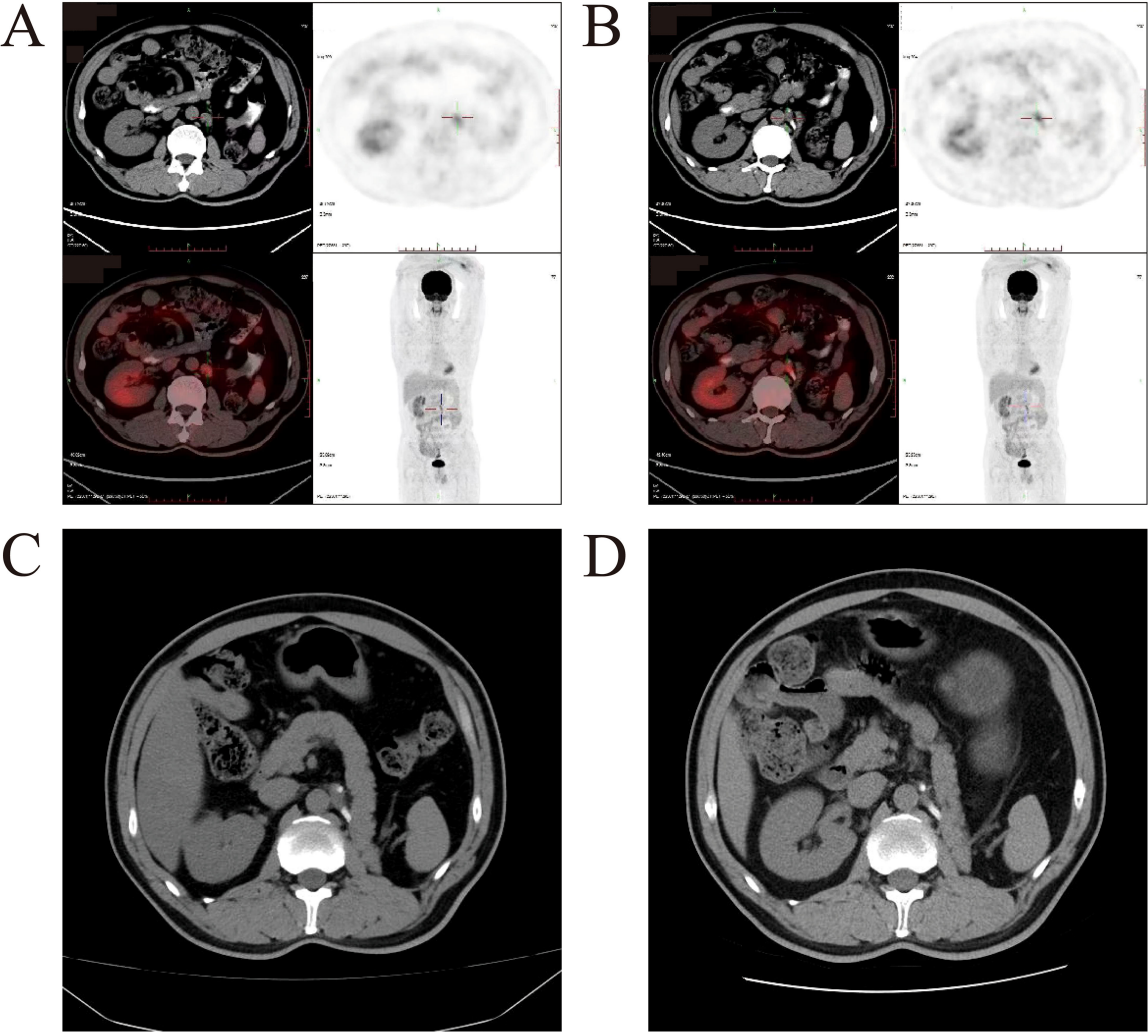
Imageological examination before and after combination therapy with toripalimab and axitinib. (A, B) Representative PET-CT images of patient before therapy. Enlarged lymph nodes were confirmed as tumor metastases by PET-CT. (C) Pre-treatment image shows retroperitoneal lymphadenopathy (white arrows). (D) Post-treatment image demonstrates significant reduction in the size of metastatic lymph nodes (white arrows), consistent with a partial response to therapy.
